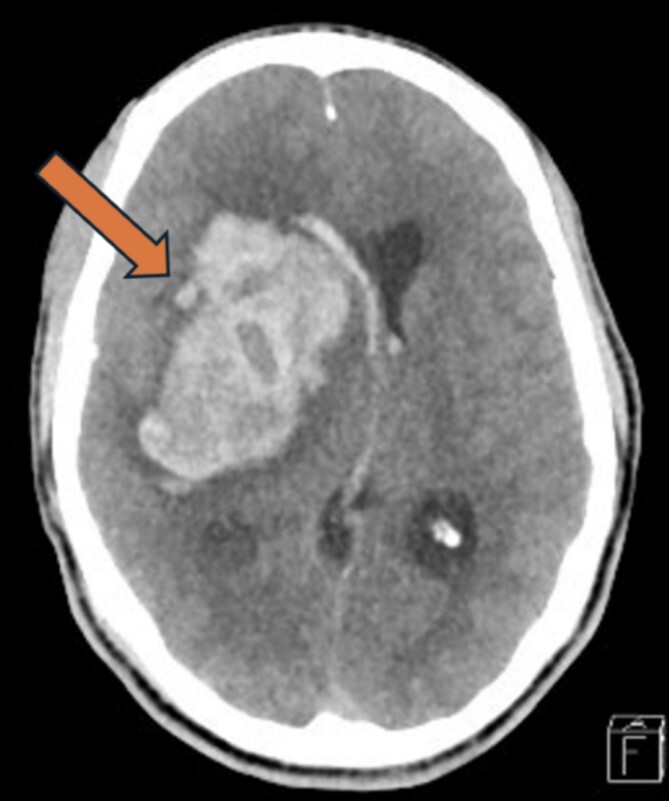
Head CT-Scan showing large intraparenchymal hematoma involving the right basal ganglia and temporal lobe with surrounding edema, extending into the lateral, third, and fourth ventricles, causing a midline shift of 13 mm with effacement of the right lateral ventricle and adjacent sulci.
